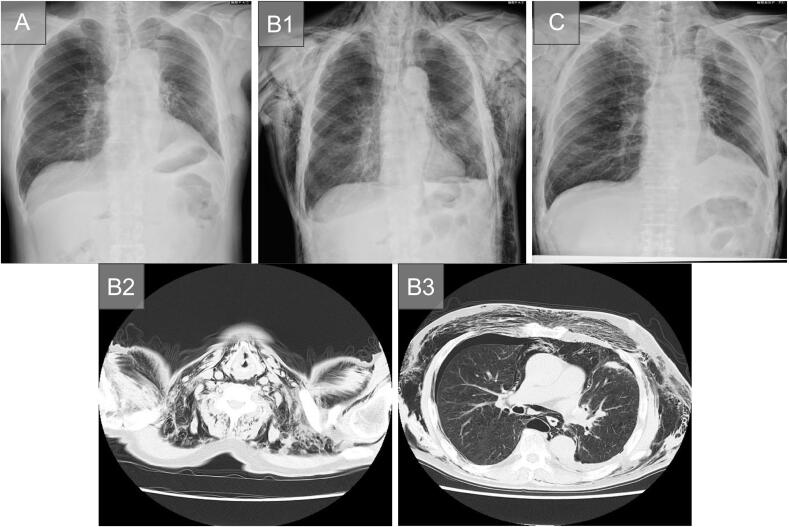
(Case 2) (A) Chest X-ray shows no subcutaneous emphysema prior to discharge (POD6). (B1) Chest X-ray at readmission shows subcutaneous and mediastinal emphysema (POD7). A slight suspicion of right pneumothorax. Chest CT at readmission shows subcutaneous and mediastinal emphysema from the neck (B2) to the chest (B3) (POD7). A slight suspicion of right pneumothorax. (C) Chest X-ray shows a reduction in subcutaneous emphysema compared to that observed prior to discharge (POD22). Abbreviations: CT: computed tomography; POD: postoperative day.
